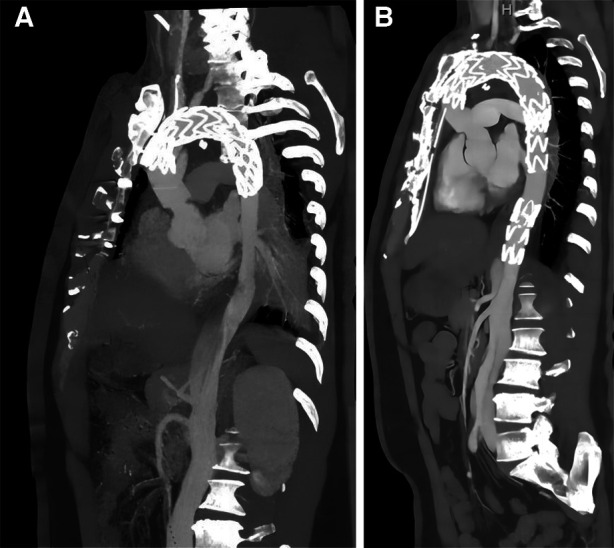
Comparison of EVAR before and after surgery by CTA. The second surgery underwent EVAR (a anteriorly and b posteriorly) with good results and significant improvement in true vena cava flow, but with residual abdominal aortic dissection, possibly facing reoperation.
